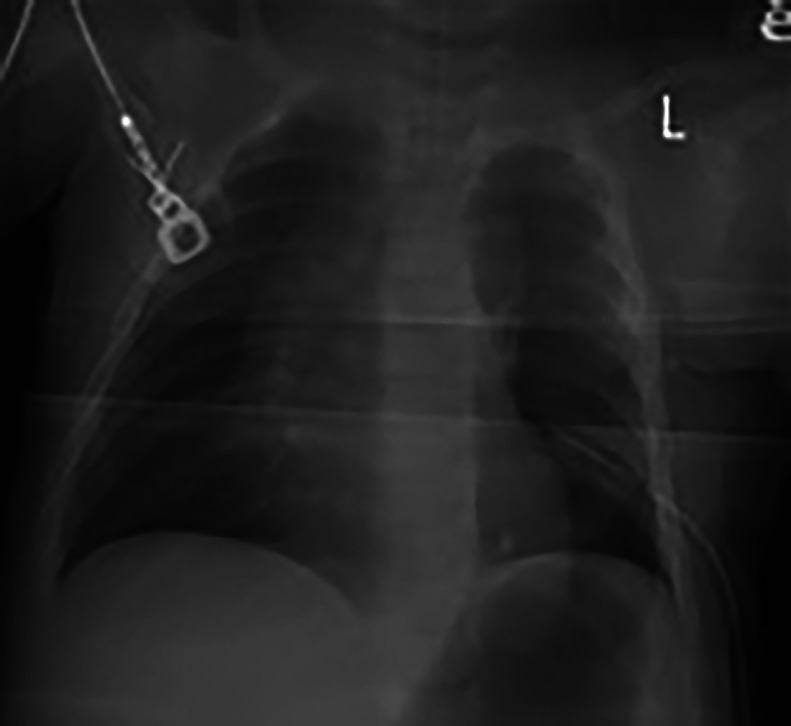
Postoperative radiograph of the neonatal chest demonstrating air-filled left lung. Note chest tube and surgical clips.
